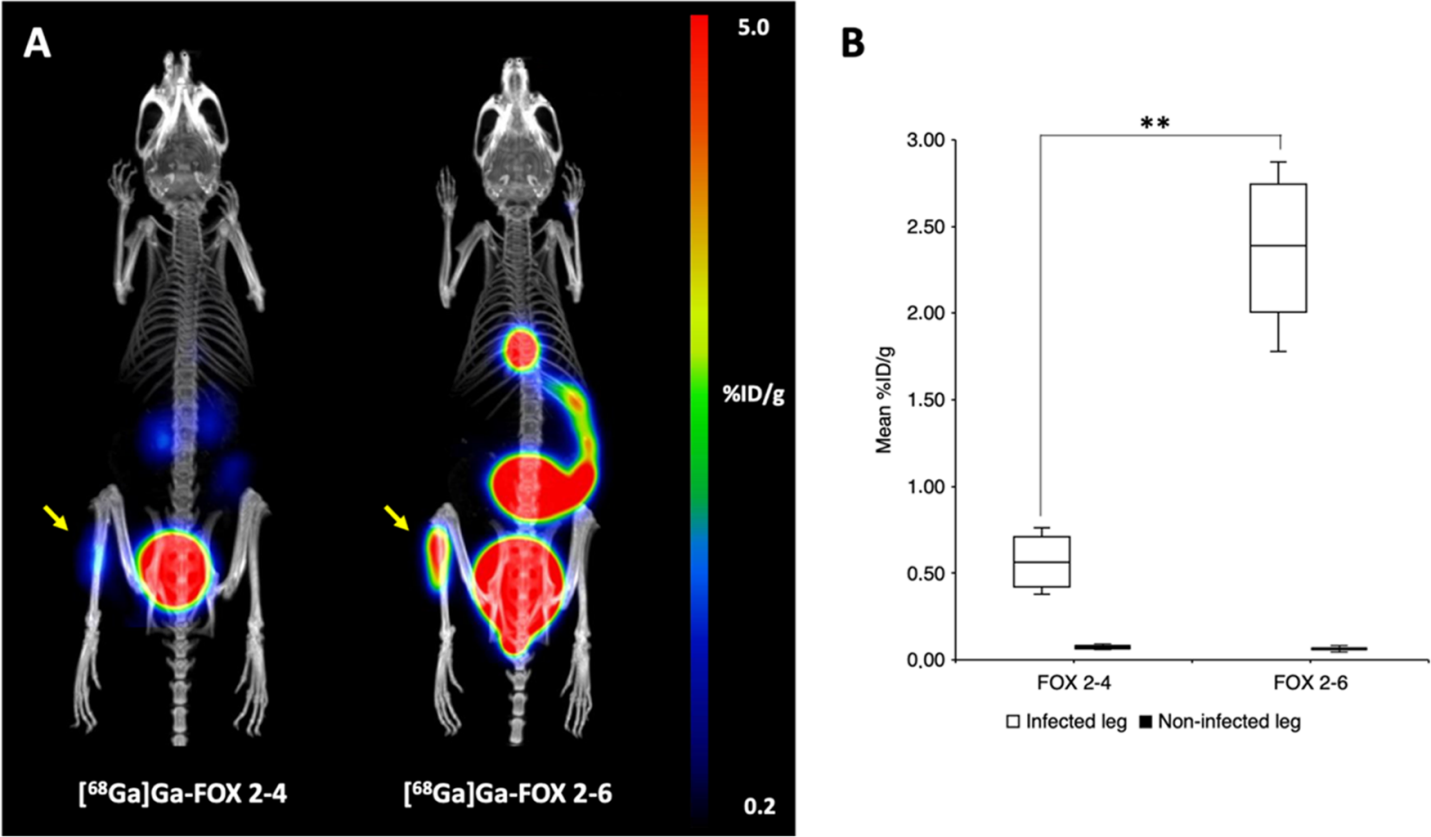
(A) Static PET/CT imaging
